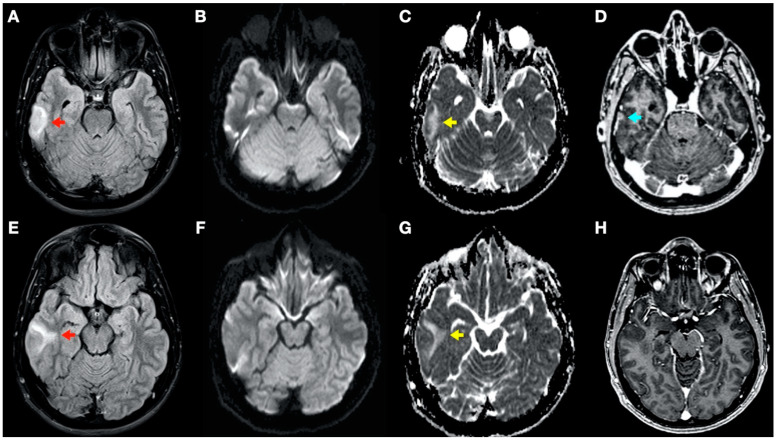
Brain magnetic resonance imaging (MRI): Images (A–C,E–G) from MRI 4 days after clinical onset; images (D,H) from MRI 5 days after clinical onset. Axial fluid-attenuated inversion recovery (FLAIR) images (A,E) show a right temporal cortico-subcortical hyperintense lesion with slight mass effect (red arrows). Axial diffusion-weighted imaging (DWI) sequences (B,F) did not show a high-signal intensity of lesions, whereas axial apparent diffusion coefficient maps (C,G), show a hyperintense lesion in this location (yellow arrows). (D,H) Axial gadolinium-enhanced 3D T1-weighted imaging (Gd T1WI) shows the presence of a slight enhancement of irregular morphology (blue arrow).
